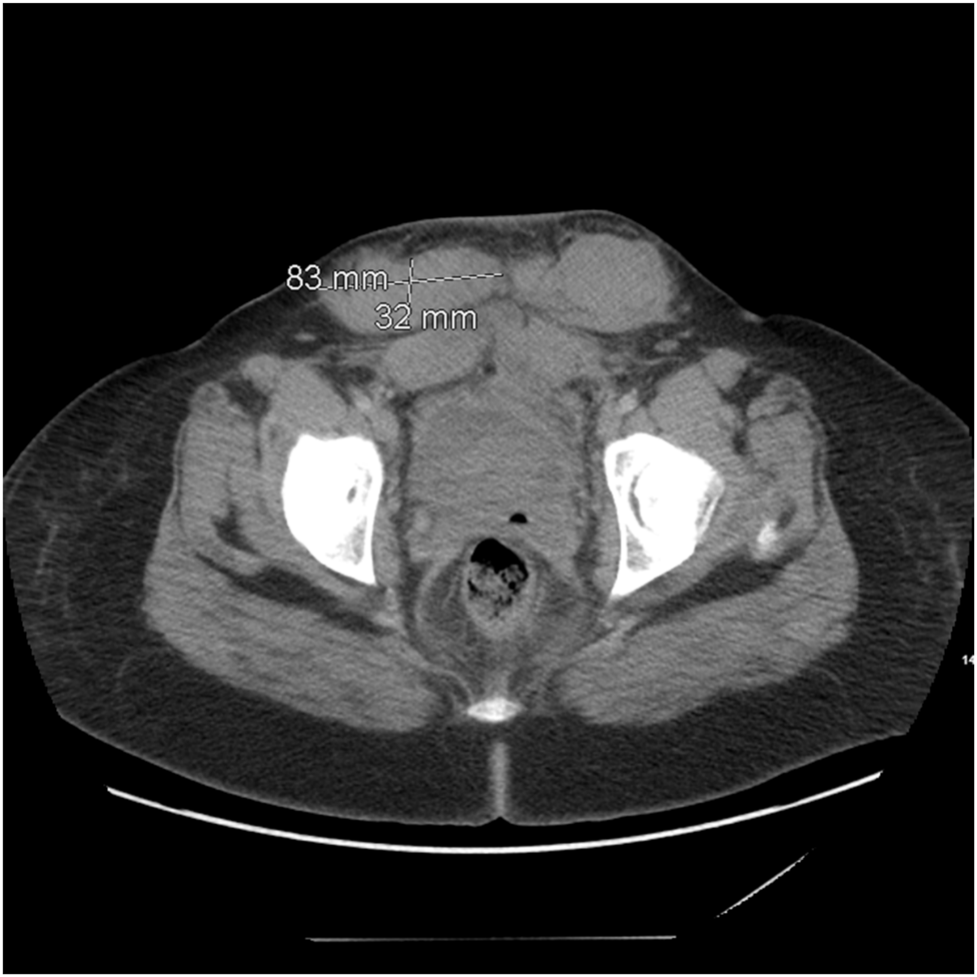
Postoperative day nine CT scan of the abdomen and pelvis following cesarean delivery. Two subcutaneous hematomas visible anterior to the lower rectus musculature at the cesarean skin incision site.
